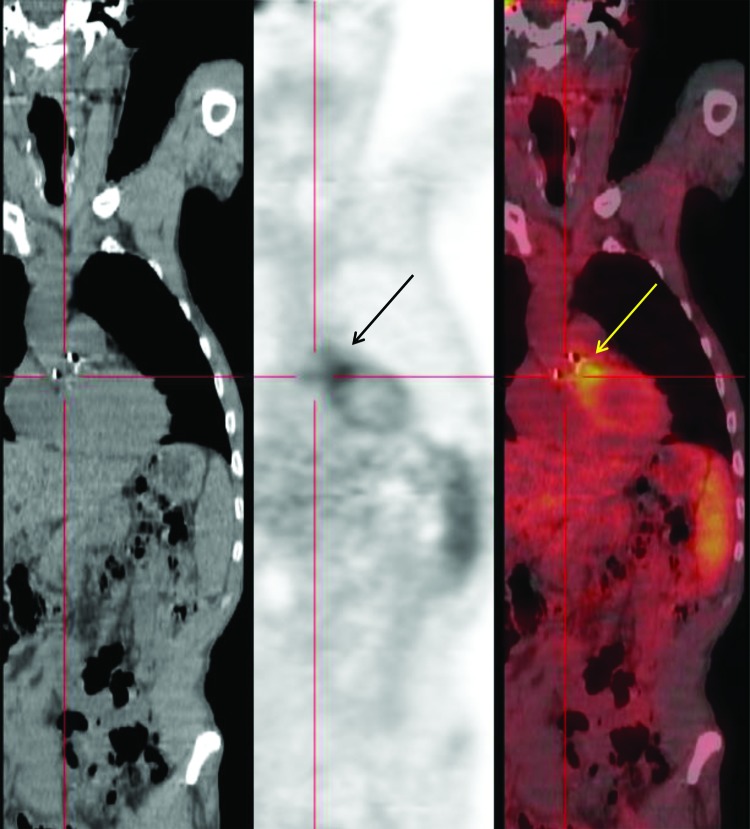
Positron emission tomography/computed tomography fusion imaging for a 56-year-old man in southern France with *Bartonella henselae* prosthetic valve endocarditis. Left panel, frontal computed tomography image showing morphologic findings. Middle panel, ^18^F-fluorodeoxyglucose–positron emission tomography (^18^FDG-PET) showing a cardiac hotspot (arrow) in relation to abnormal uptake of ^18^FDG. Right panel, fusion image combining ^18^FDG-PET and computed tomography showing localization of an aortic valve periprosthetic infection (arrow).
